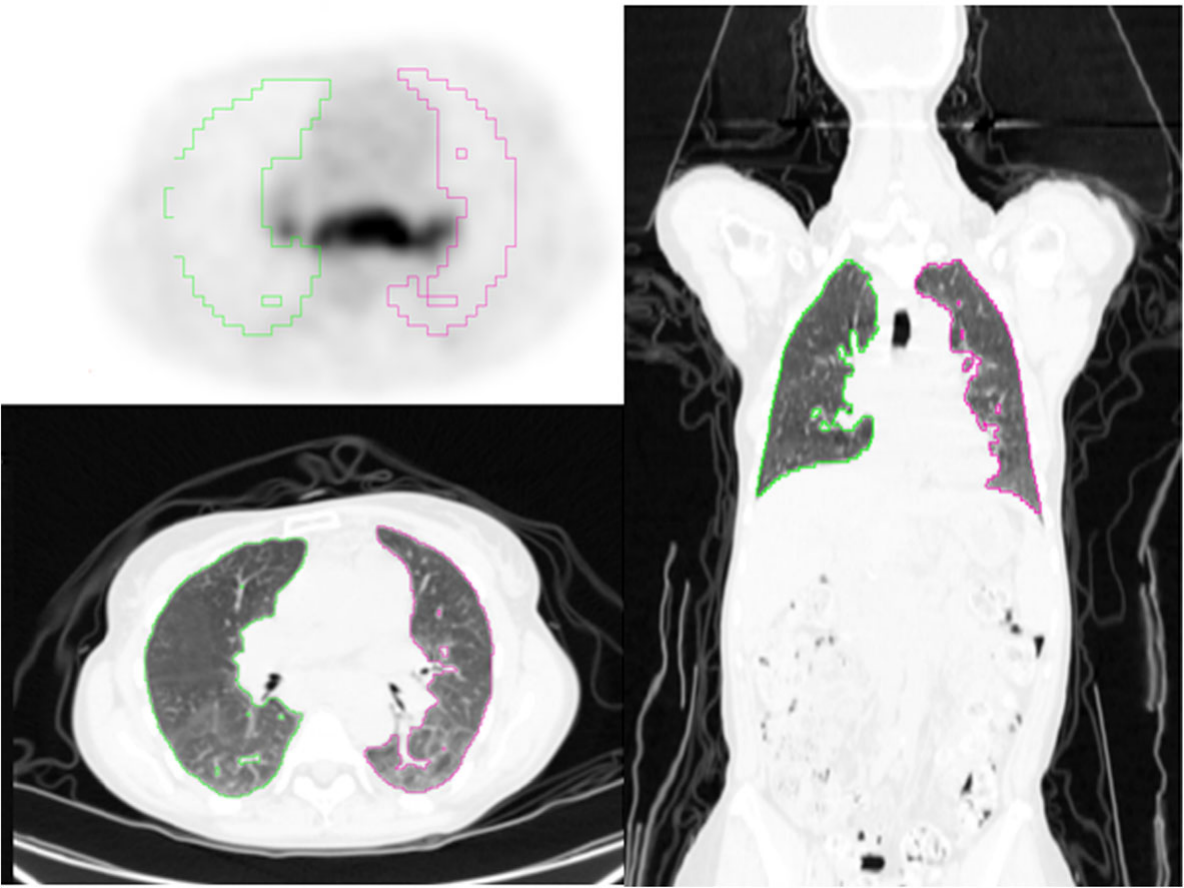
Example of VOI of total lung glycolysis. TLuG is the cumulative metabolic activity in the total lung parenchyma. Additionally, SUVmax and SUVmean are determined in the VOI
